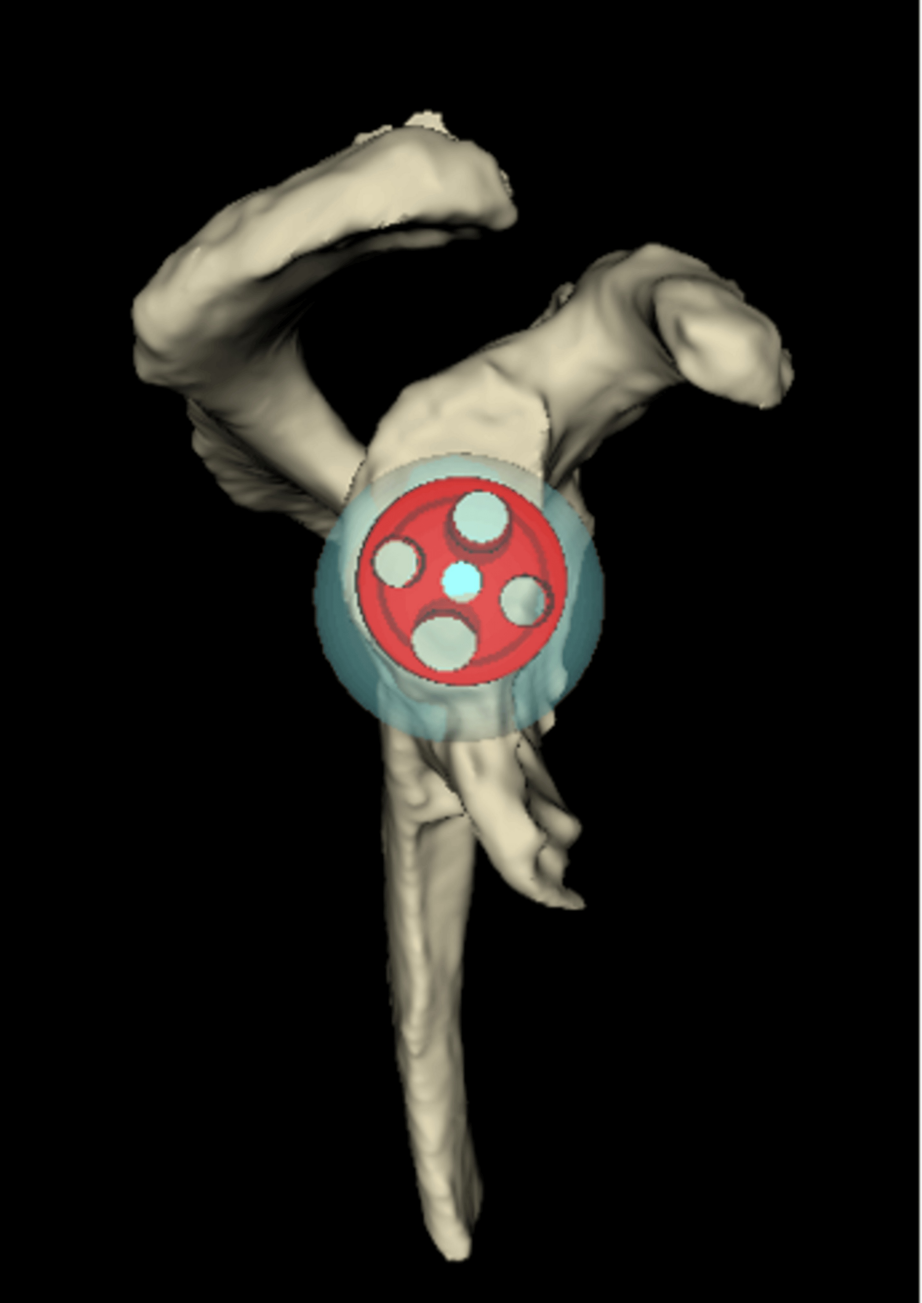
Sagittal 3D CT-based planning view demonstrating planned positioning of a size 24 glenoid baseplate and 36 mm eccentric glenosphere, allowing secure central and peripheral screw fixation despite anterior glenoid bone loss.
